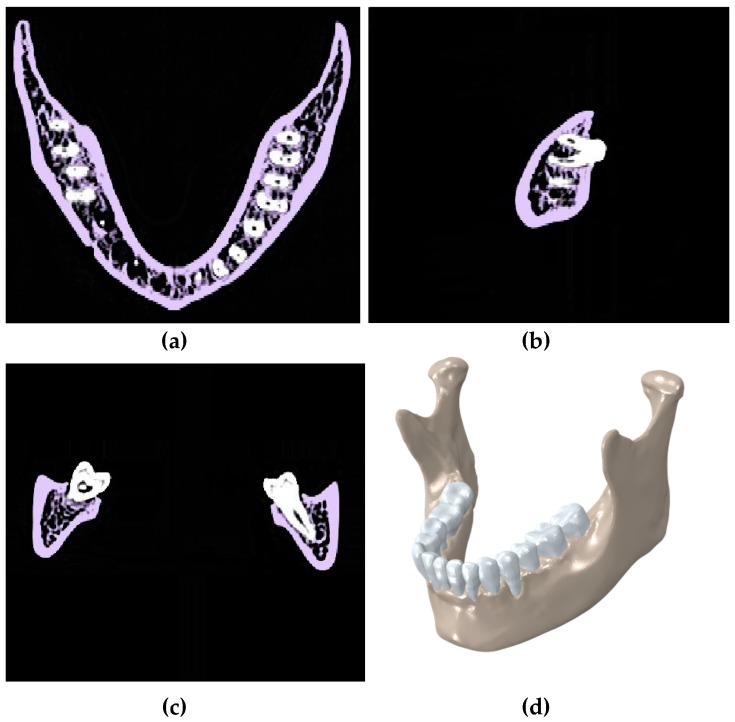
Segmentation of CT scan of a healthy mandible showing the (a) transverse plane; (b) sagittal plane; and (c) coronal plane; (d) a 3D model in Mimics.
